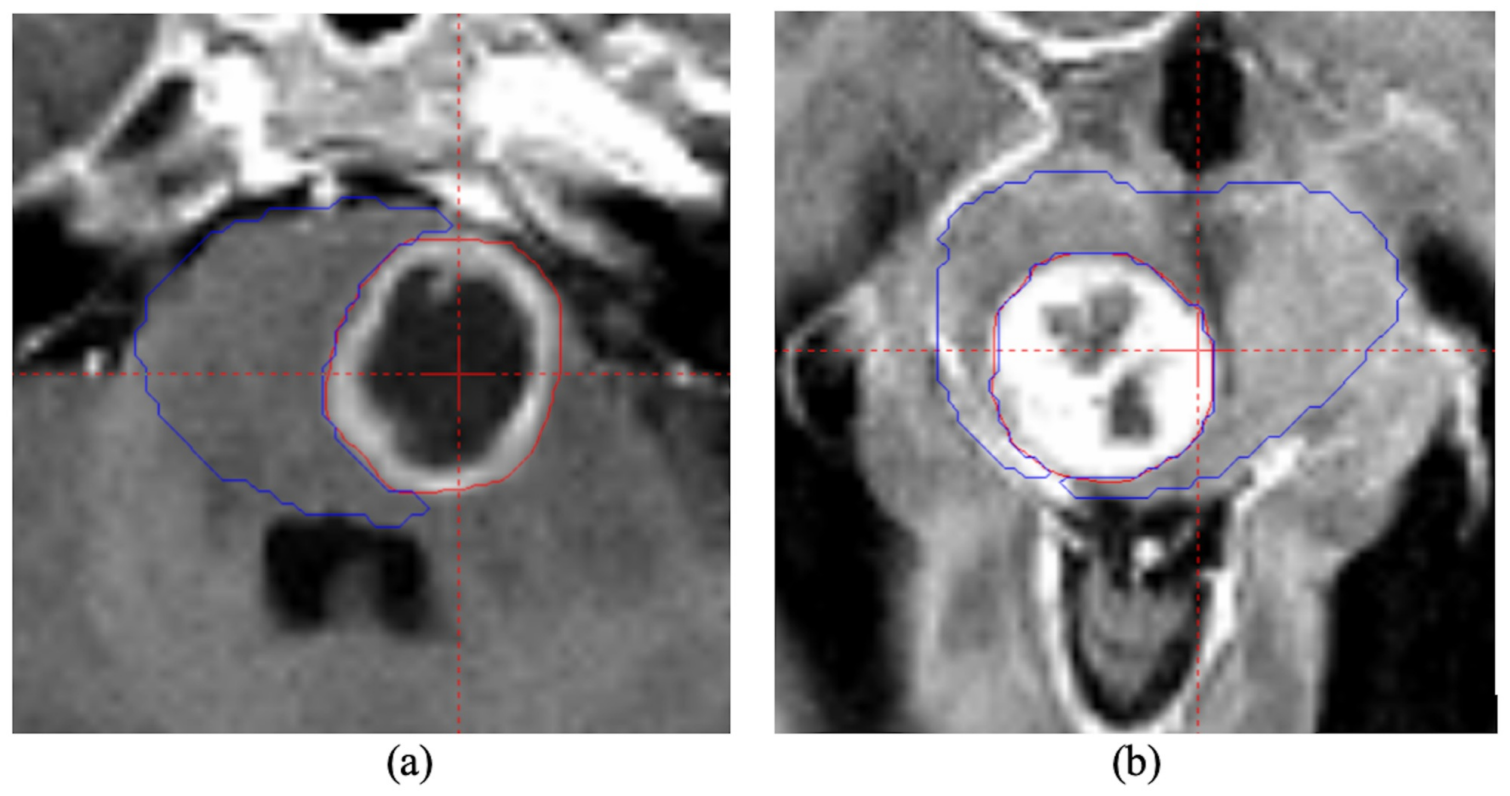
The axial slice of T1-weighted Magnetization Prepared RApid Gradient-Echo (MP RAGE) MR image post contrast for two patients. The red line indicated the tumor drawn by the physician and the blue line was the brain stem. They were the patient 3 (a) and 5 (b) in Table 1.
